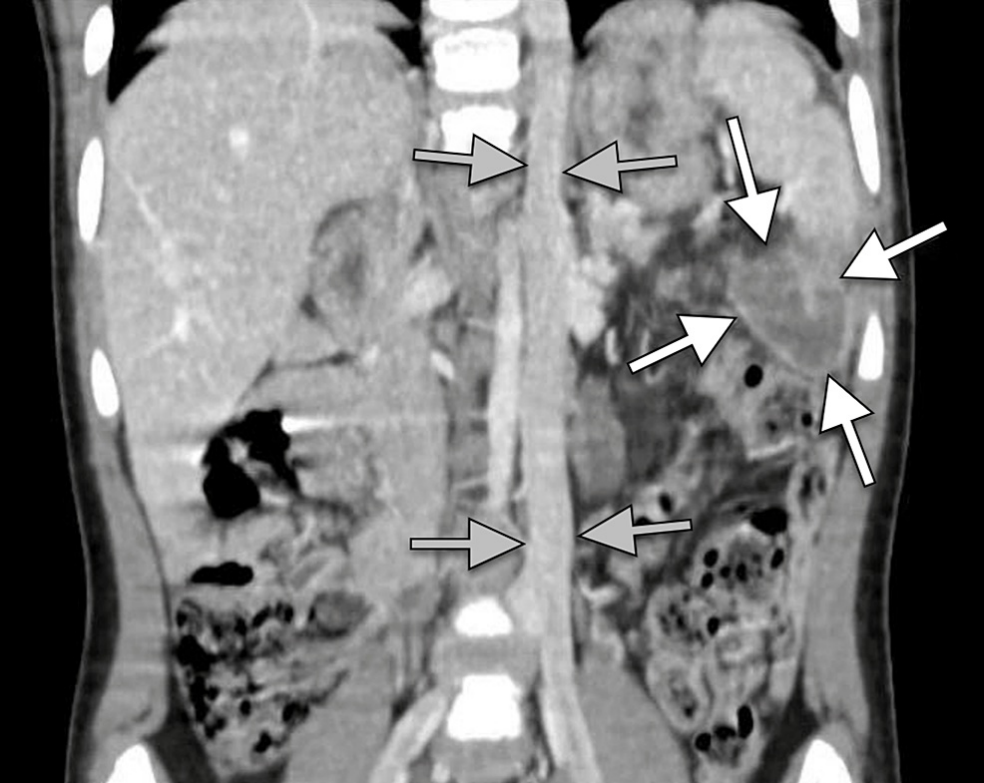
Coronal CT image of the abdomen presenting a homogeneous hypodense mass with an enhancing wall (white arrows) and the continuation of the hemiazygos vein as a left-sided inferior vena cava (gray arrows). CT, computed tomography.
